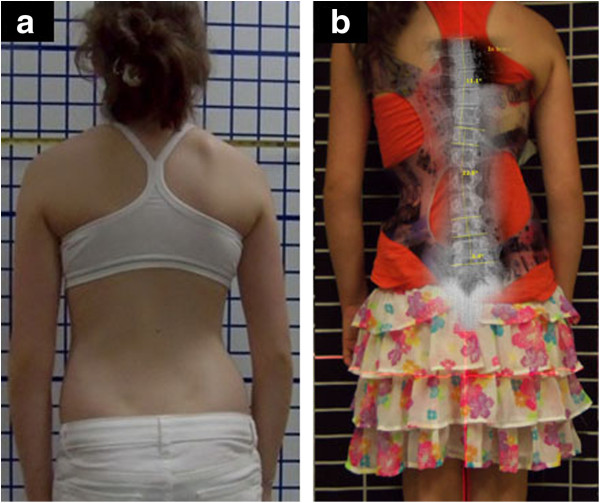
WCR brace with improved clinical presentation of patient. a: The clinical presentation of the patient with a right thoracic curve of 22-degree Cobb angle and a left lumbar curve of 22-degree Cobb angle. b: X-ray of patient in the Cheneau-Rigo handmade type brace showed over 50% correction of scoliosis, which improved from thoracic 22 degrees Cobb and lumbar 22 degrees Cobb out-of-brace to thoracic 11 degrees Cobb and lumbar 10 degrees Cobb in-brace.
